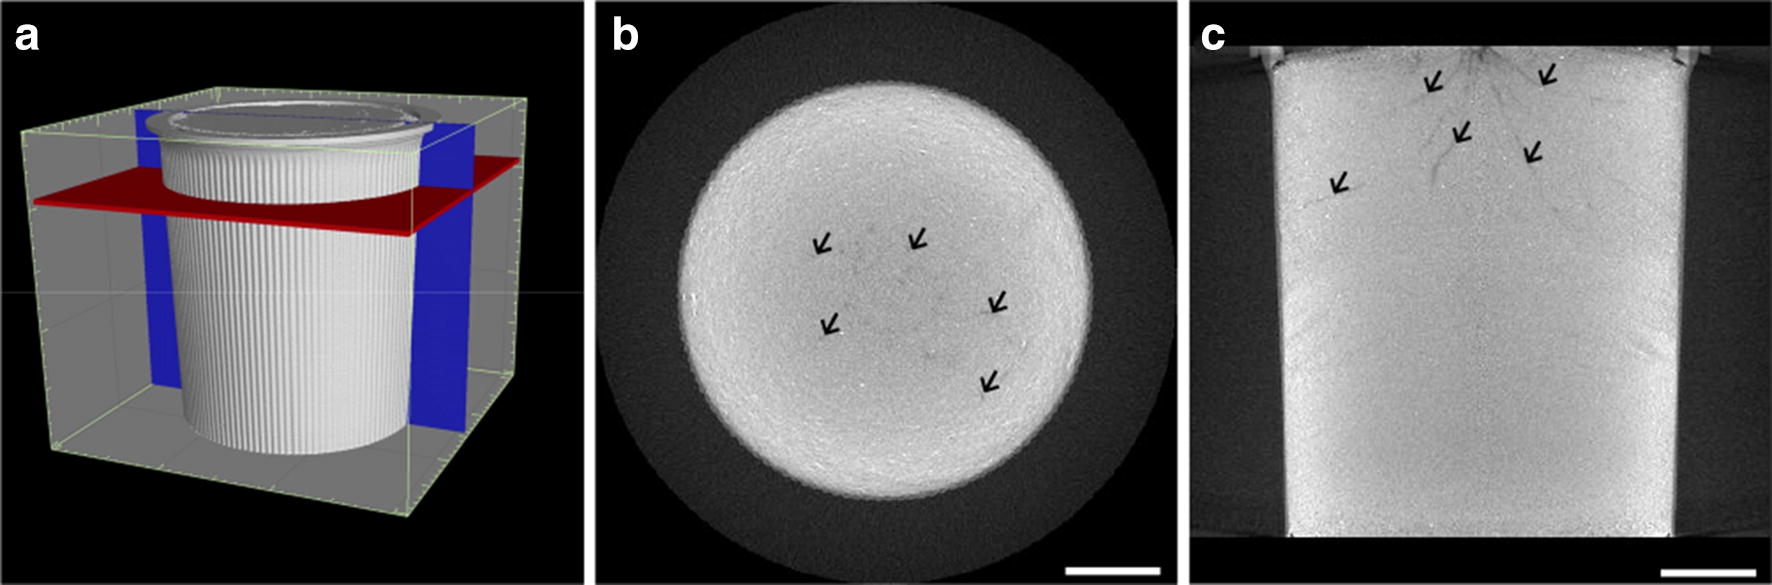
Representative X-ray CT images of a pot filled with calcined clay, using a 4-week-old Kinandang Patong as the scanning subject: a volume rendering with X-ray CT volume. Scale interval is 1.0 cm; b horizontal; and c vertical slices of X-ray CT volume, whose positions are indicated with red and blue rectangles, respectively, in a. Representative root fragments in b and c are indicated by arrowheads. Scale bar length: 5 cm
